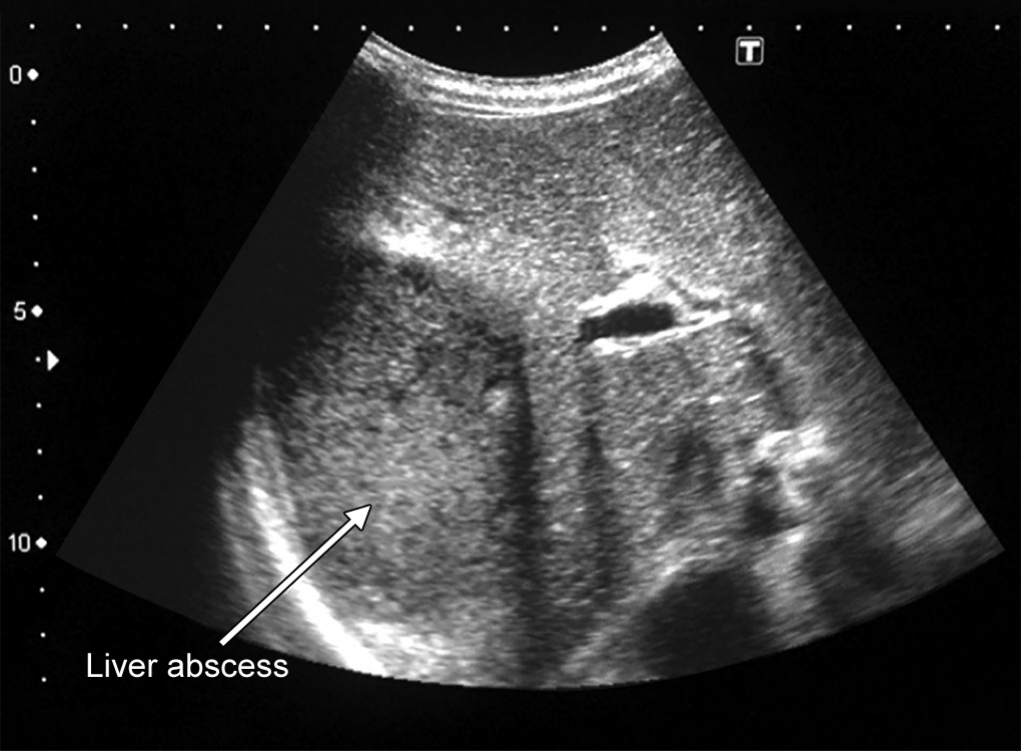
Ultrasonography showing abscess in liver
